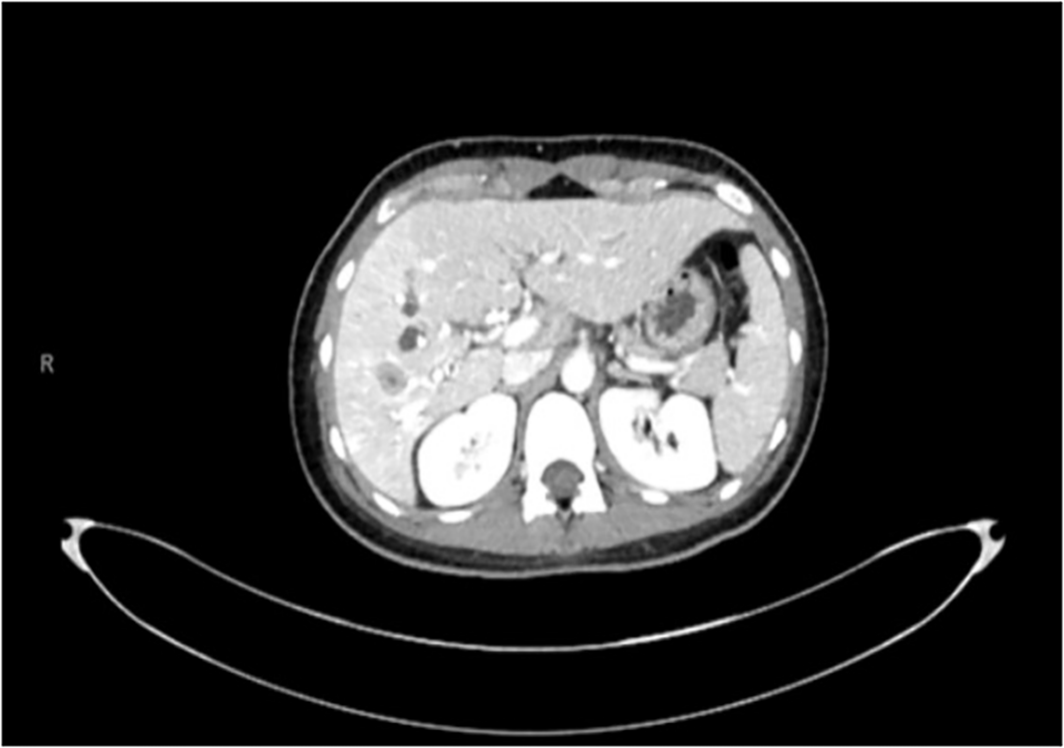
Initial CT scan dated July 2021 showcasing hypodense lesions on segment V of the liver.
